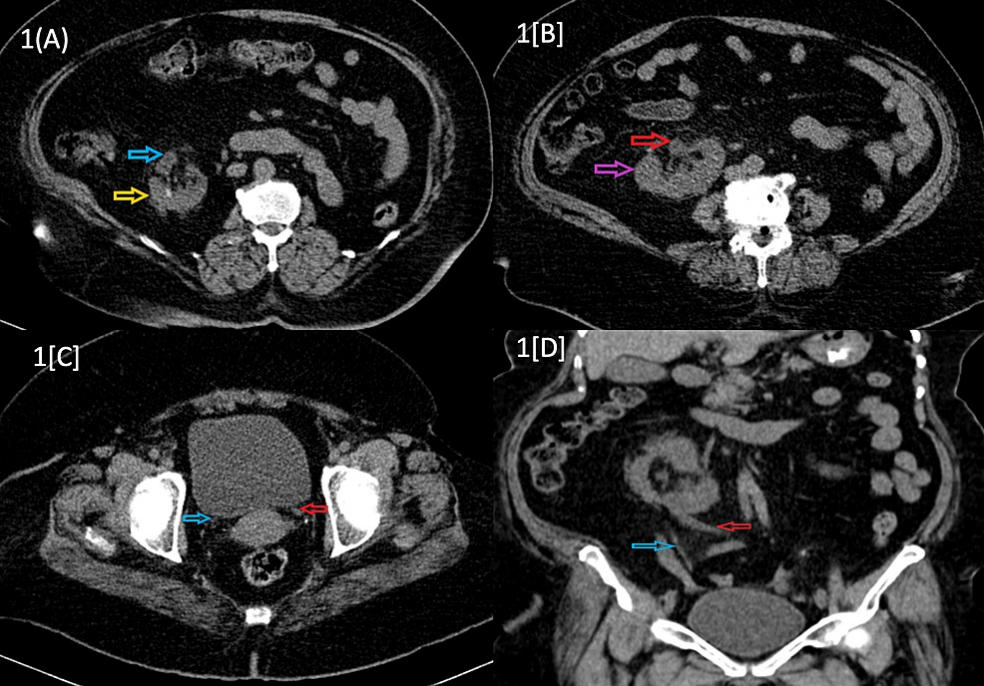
(A) CT axial section showing right kidney in right renal fossa (yellow arrow) with pelvis rotated anteriorly (blue arrow). (B) CT axial section showing left kidney in the lumbar region (purple arrow) with pelvis rotated anterolateral (red arrow). (C) CT axial section showing left ureter (red arrow) and right ureter (blue arrow) normal opening in the trigone of the bladder. (D) CT coronal section showing left ureter (red arrow) crossing midline and right ureter (blue arrow) is following its normal course
